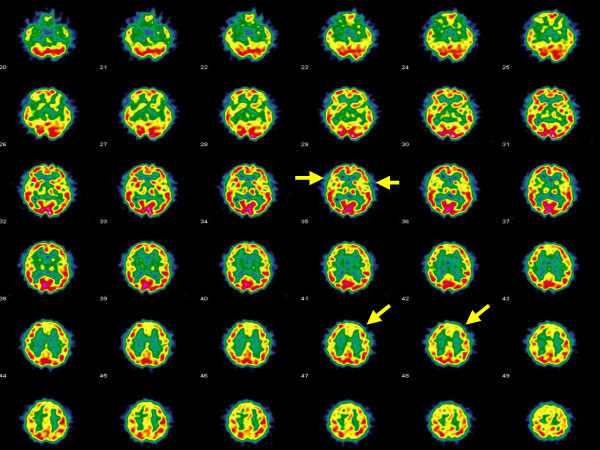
Brain SPECT transaxial images showing diffuse patchy cortical distribution of 99 mTc-ECD, in particular significant cortical hypoperfusion in the left frontal lobe and in both temporal lobes (arrows).
